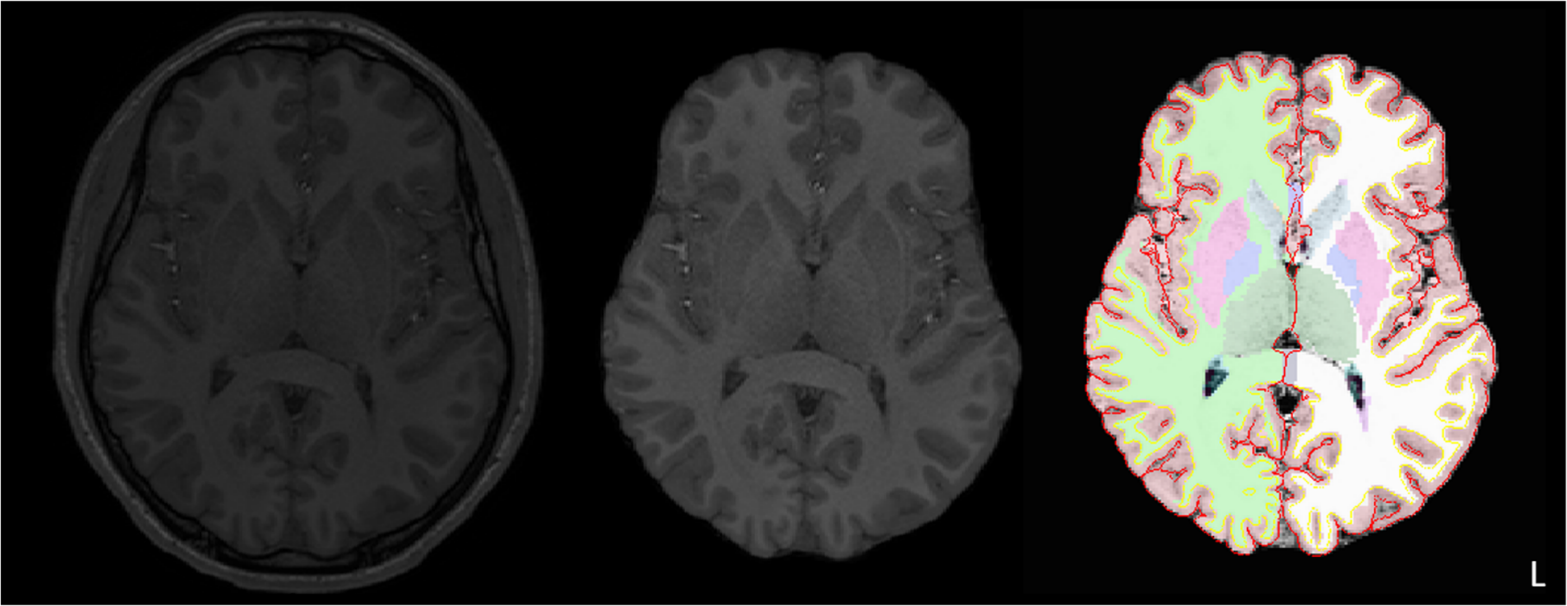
Raw T1-weighted images (left) were N3 bias corrected and skull-stripped, with manual corrections to ensure cortex was not removed (middle). Skull-stripped images were processed with Freesurfer with manual corrections (right), producing volume estimates
